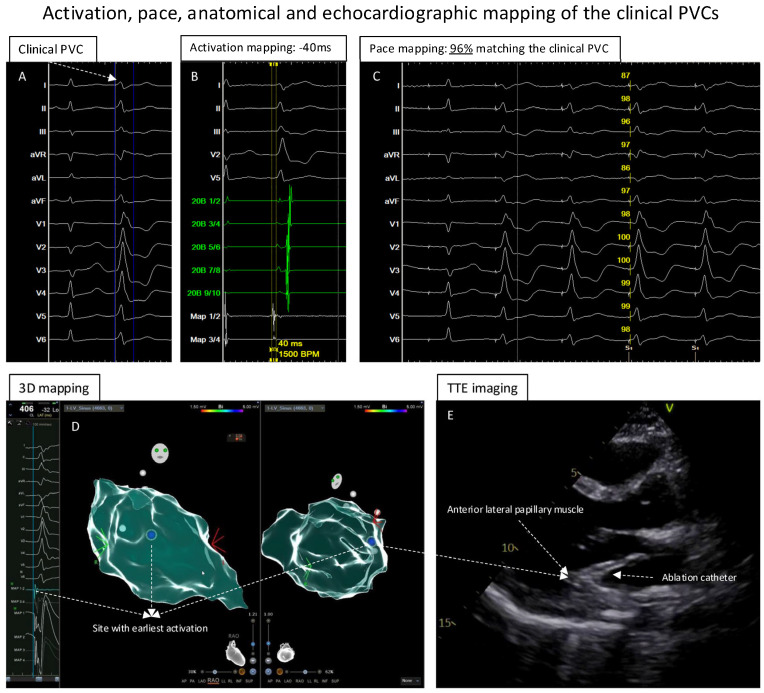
Activation, pace, anatomical and echocardiographic mapping of clinical PVCs. (A): The clinical PVC documented in the template. (B): The activation mapping of the PVCs showed the earliest local activation with timing of −40 ms before the QRS of the PVCs. (C): At the earliest activation site, the pace-mapping technique showed 96% matching score of the clinical PVCs. (D): Electroanatomic mapping of the PVCs in LV, the blue point presented the site with earliest activation and excellent unipolar electrograms. (E): While mapping the earliest activation site, TTE showed that the catheter was positioned at the anterior–lateral papillary muscle of the LV.
